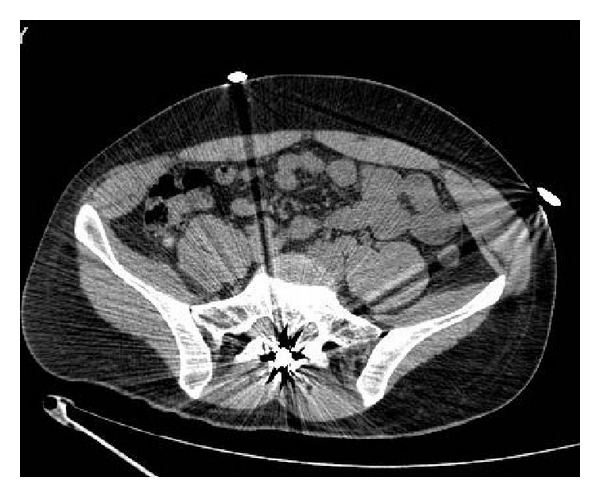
Axial CT scan demonstrates the intracanal position of the bullet and no damage to the posterior arc of S1-S2 level.
